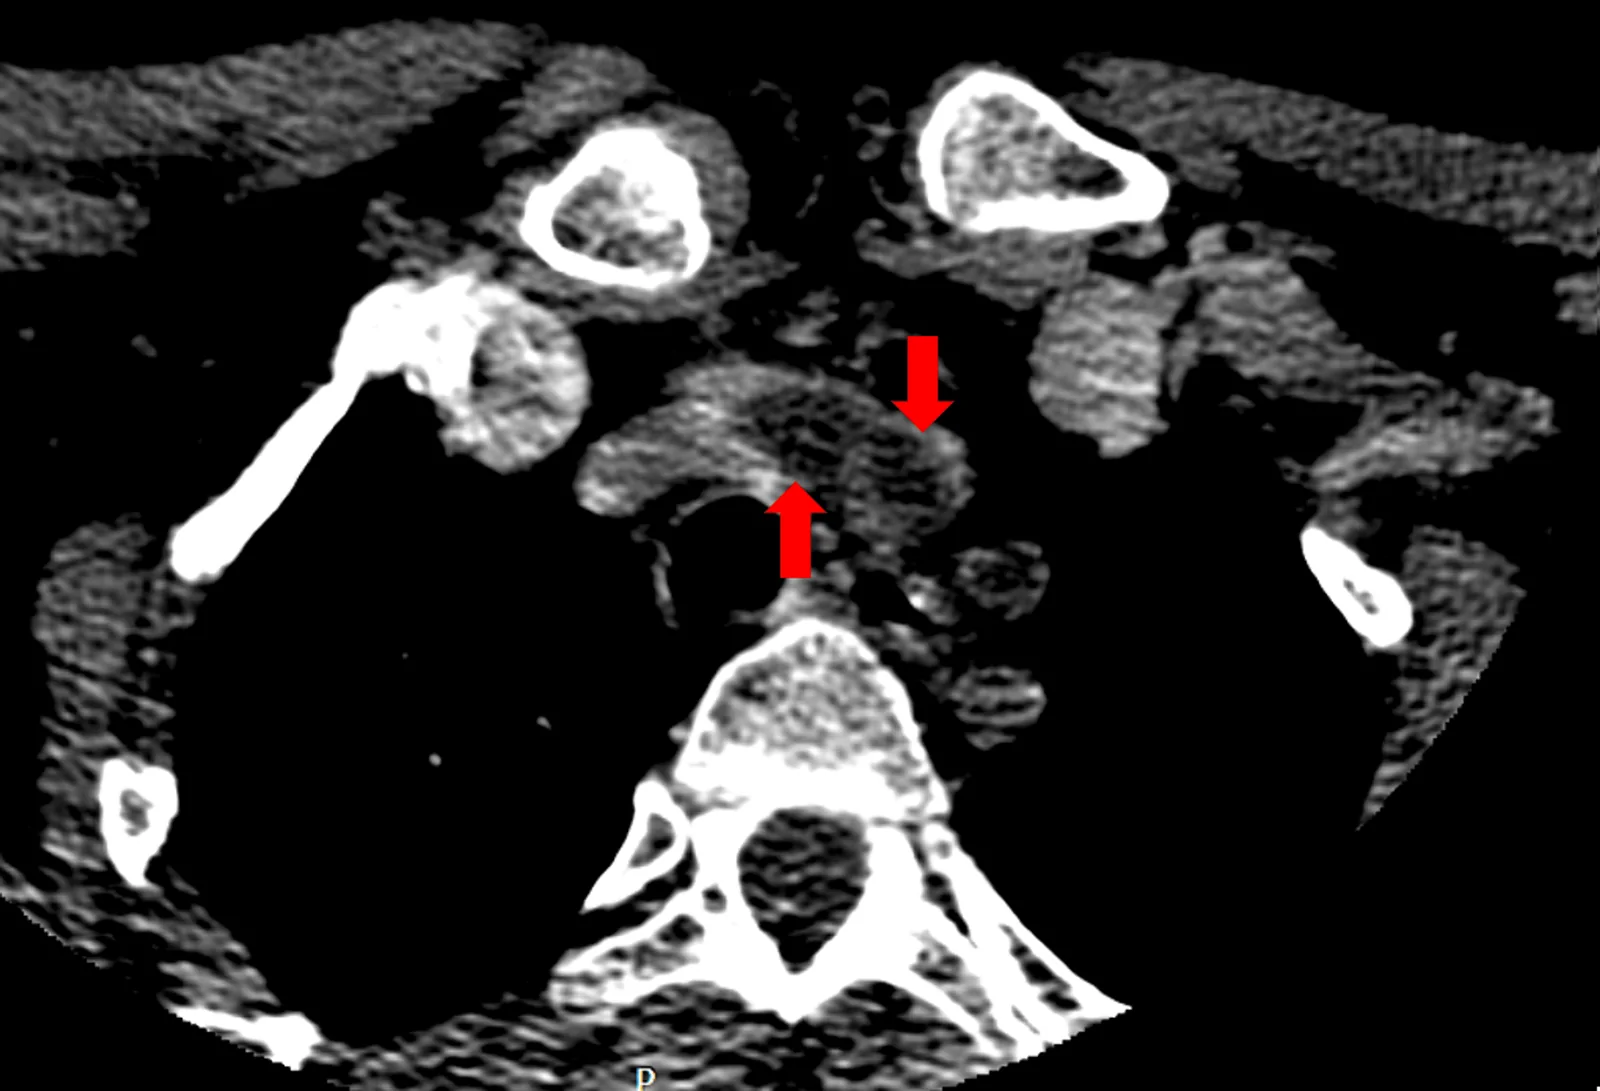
CTA showing a floating aortic thrombus extending to the brachiocephalic arterial trunk and the left common carotid artery in a 50-year-old female patient (Case 2)
